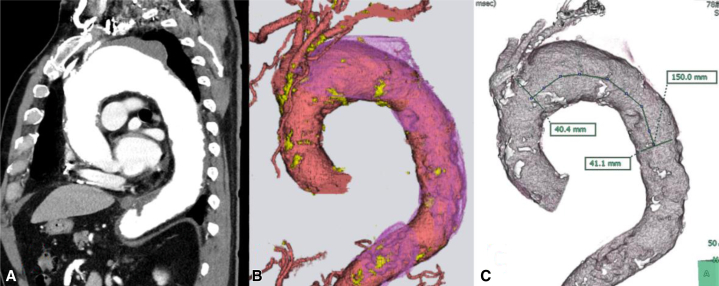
Preoperative findings of Figure 2. A, Contrast-enhanced computed tomography (*CT*) revealed a saccular distal aortic arch aneurysm measuring 64 mm in diameter and a fusiform descending aortic dilatation measuring 42.6 mm in diameter. B, Three-dimensional CT of the aneurysm. C, Measurement of the aortic diameter and length on 3-dimensional CT. Even with the use of a 150-mm stent graft of the Thoraflex Hybrid prosthesis, the descending aortic landing zone measured 41.1 mm in diameter, exceeding the applicable size for the device.
